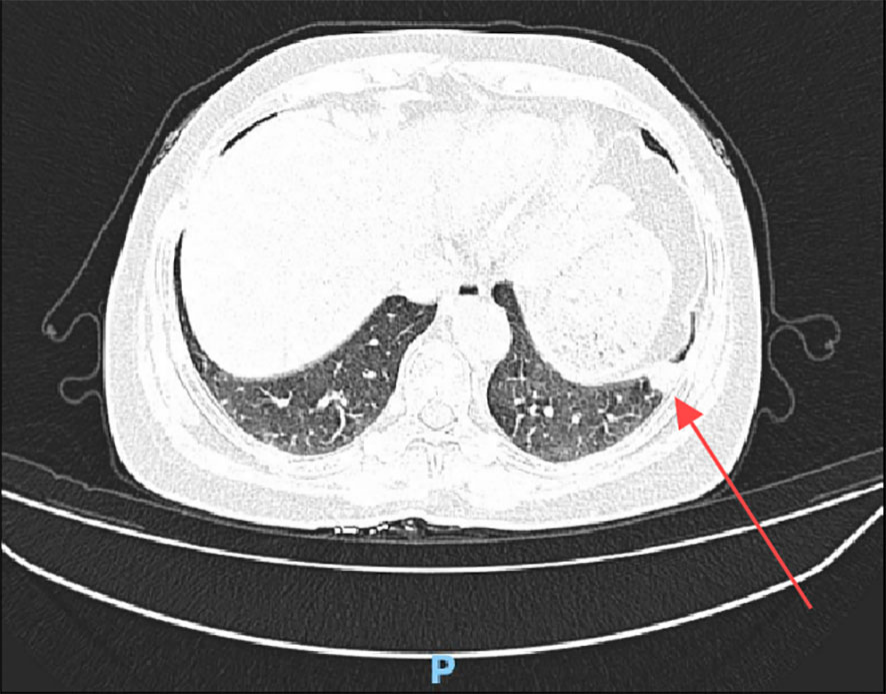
Chest CT shows a high-density nodule in the outer basal segment of the left lower lobe (red arrow).
